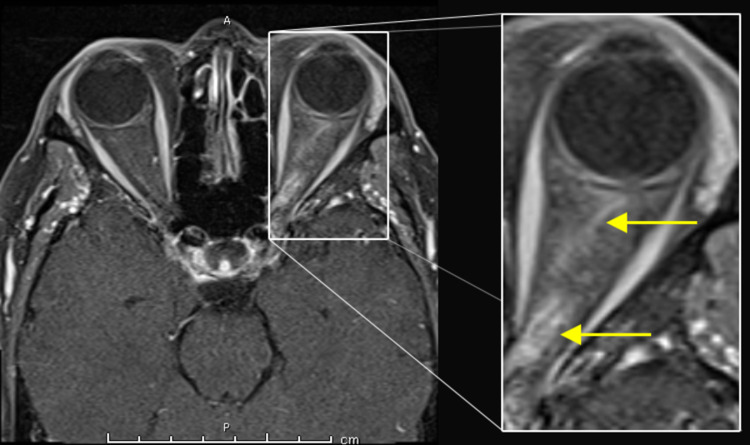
T1-weighted axial MRI gadolinium-enhanced image: enhancing and thickened optic nerve (yellow arrows)
